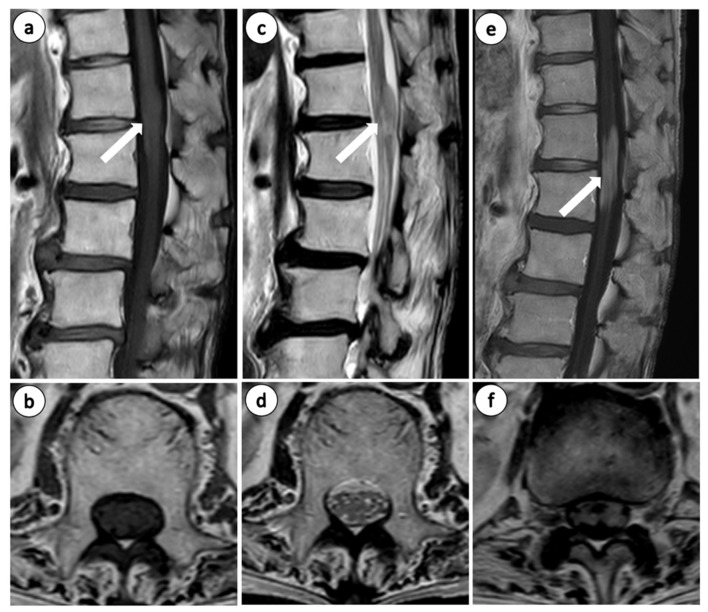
Lumbar MR images: T1WI (a) sagittal and (b) axial, and T2WI (c) sagittal and (d) axial section at the L1 level. Gadolinium-enhanced MR images: (e) sagittal and (f) axial section at the L1 level. The arrow indicates abnormal signal changes.
